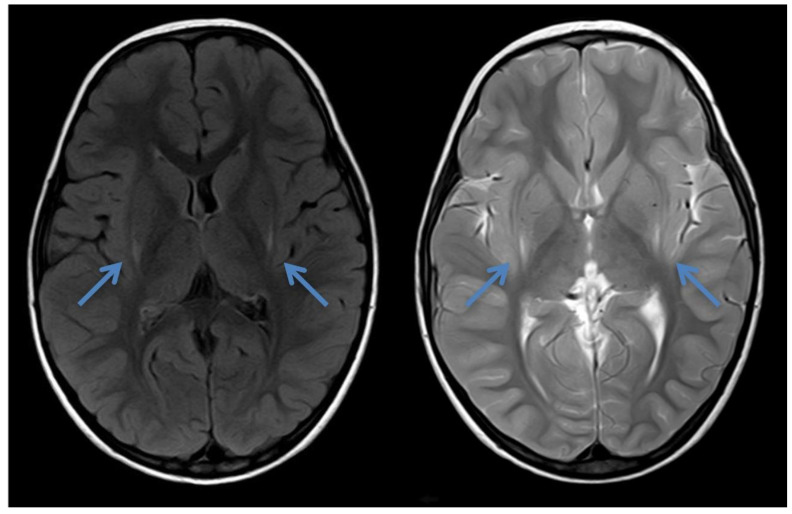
Cranial MRI of patient 2 at the age of 2 years and 8 months showing laterodorsal increased signal intensity bilaterally around the putamen (blue arrows) in axial FLAIR and T2—the typical insidious onset pattern of GA-1.
